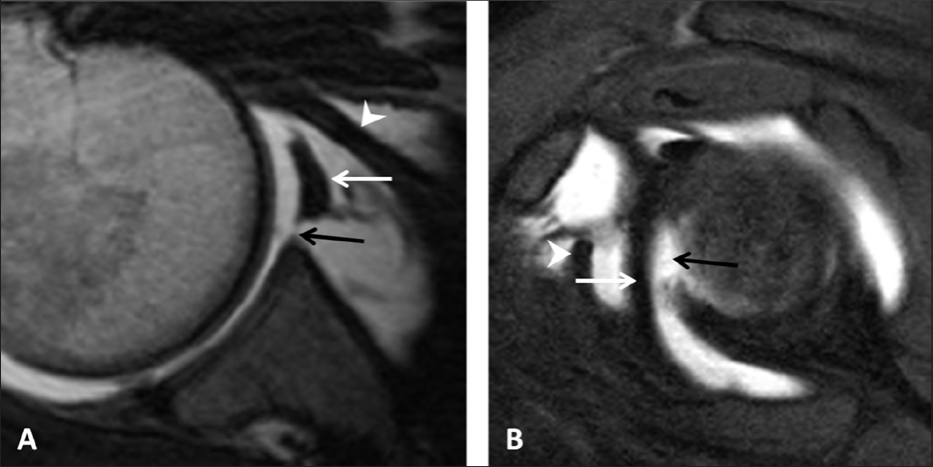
Buford complex. (A) Axial PD-weighted and (B) Sagittal fat-suppressed T1-weighted MR arthrographic images show a cord-like middle glenohumeral ligament (white arrow) associated with an absent anterior superior labrum (black arrow) mimicking a labral tear with normal posterior labrum. Subscapularis tendon (arrowhead). (Courtesy of Dr Henri Guerini).
